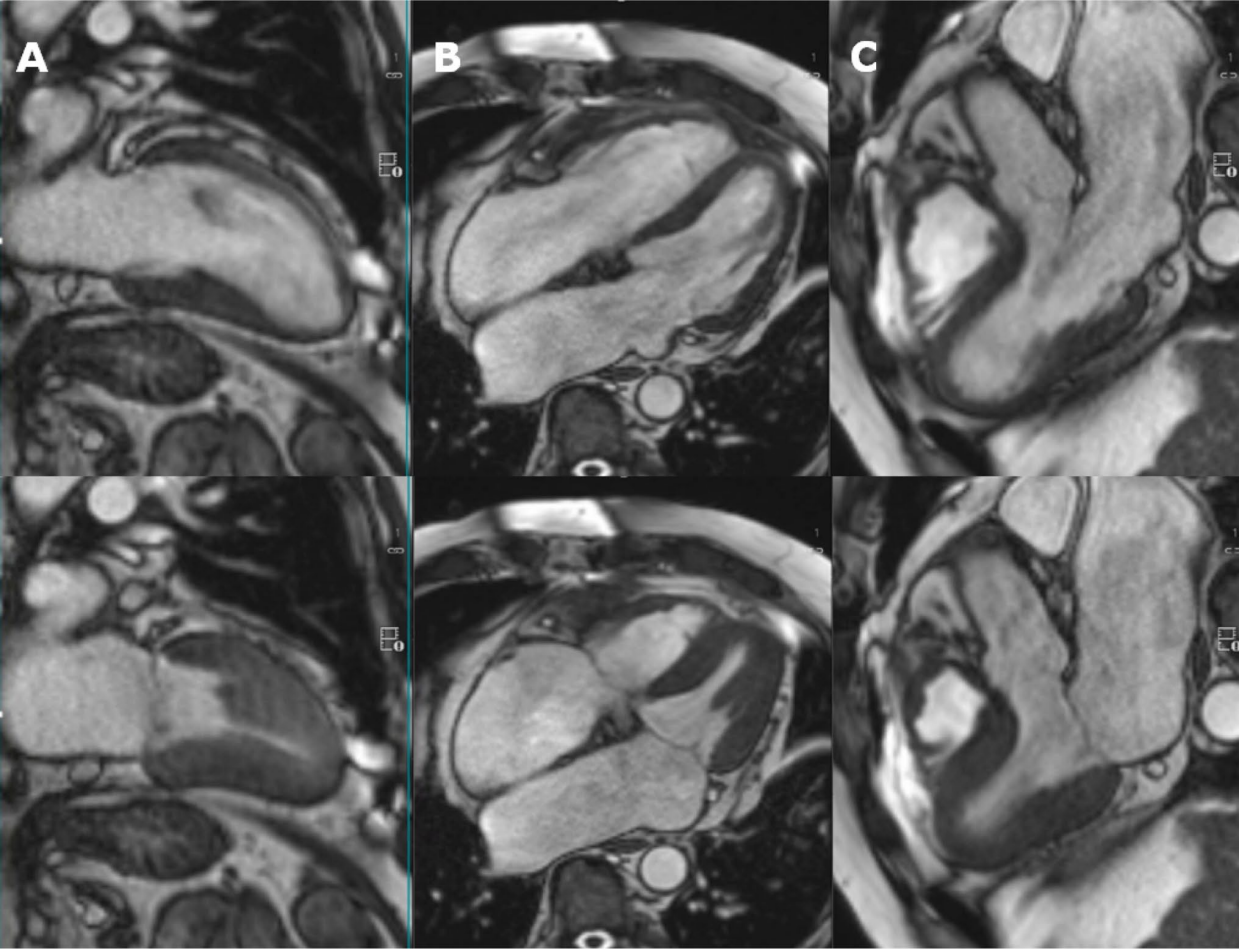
Cardiac MRI. Upper panel diastole, lower panel systole. A 2-chamber view. B 4-chamber view. C 3-chamber view. See also video 2A and B. A homogeneous, normal left ventricular contraction is seen, ejection fraction amounts to 62%. Left ventricular mass index is normal (71 g/m^2^). Combined recipient-donor atria are severely enlarged
